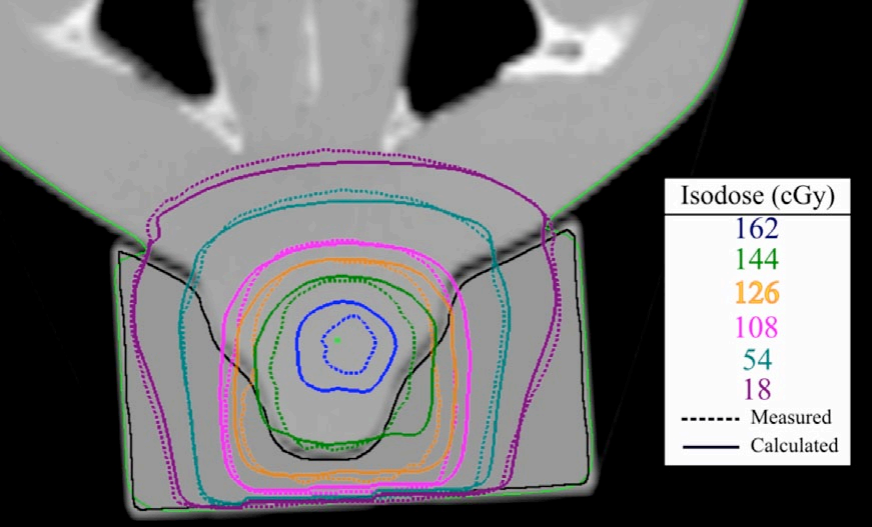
Dose plane comparison between the calculated dose plane from Eclipse RTP using a plan with a new CT with printed bolus, and the measured dose plane using Gafchromc film. 89.6% of points were passing with gamma criteria of 2 mm DTA and 5% dose difference.
